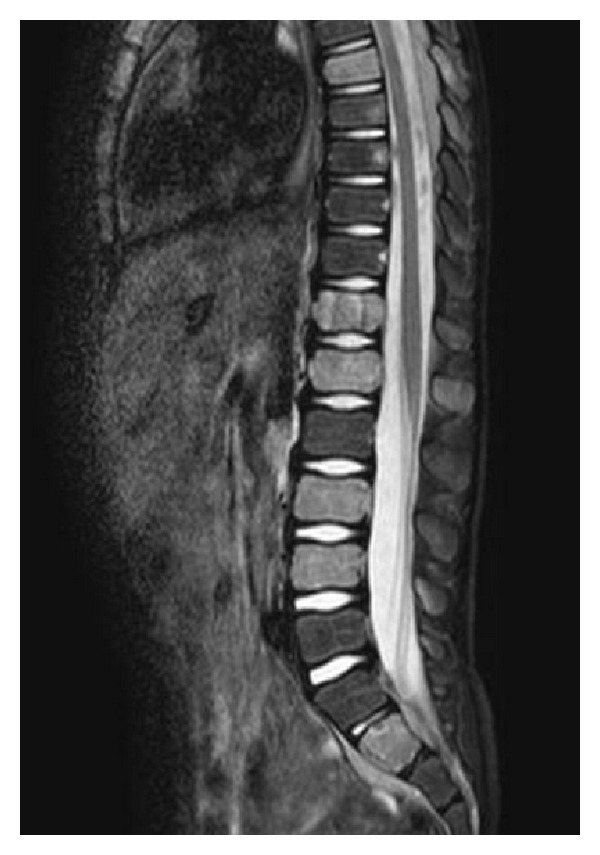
Spine MRI (median sagittal STIR): heterogeneous captation in some vertebrae (T7, T12, L1, L3, L4, S2), suggesting bone marrow infiltration.
